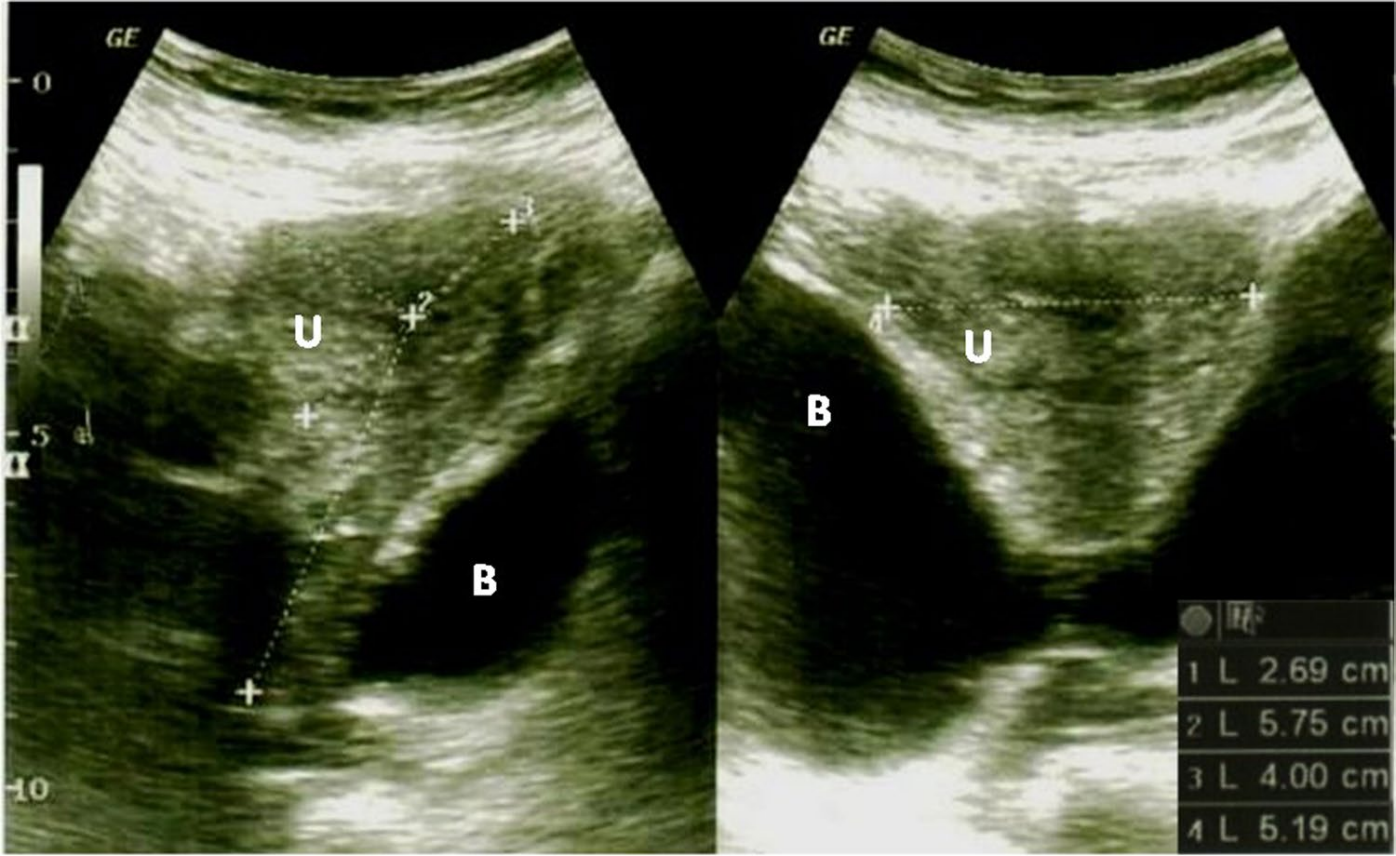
Good attachment of uterus to the abdominal wall can be observed on ultrasound after LONG suspension.
